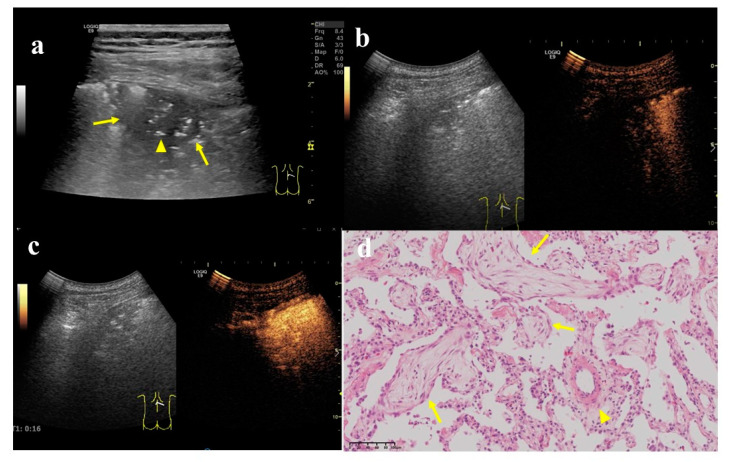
A 62-year-old female, who underwent total gastrectomy for gastric cancer 6 years ago and breast-conserving surgery for breast cancer 2 years ago, was coughing and expectorating for one month. (a) A wedge-shaped hypoechoic lesion (arrow) can be seen on the ultrasonic image of the right lung, with air bronchial sign inside (arrow head). Either angle between lesion border and thoracic wall was an acute angle. (b) After injection of contrast agent, the lesion began to enhance in 7 s, which was a uniform hyper-enhancement. The lung tissue began to enhance in 9 s, later than the lesion. (c) The enhancement of the lesion reached the peak in 16 s, and was a uniform enhancement. (d) An ultrasound-guided biopsy was performed. The pathological examination showed alveolar structure existed, the spacing was widened, and scattered lymphomonocytes could be seen in the focal alveolar cavity with cellulose exudation. Masson bodies (arrow) and small branch of pulmonary artery (arrow head) can be seen inside (×200). These findings conformed to the changes in organized pneumonia. After treatment with Medrol 8 mgqd for two months, the lesion became smaller and did not enlarge at the time of writing.
